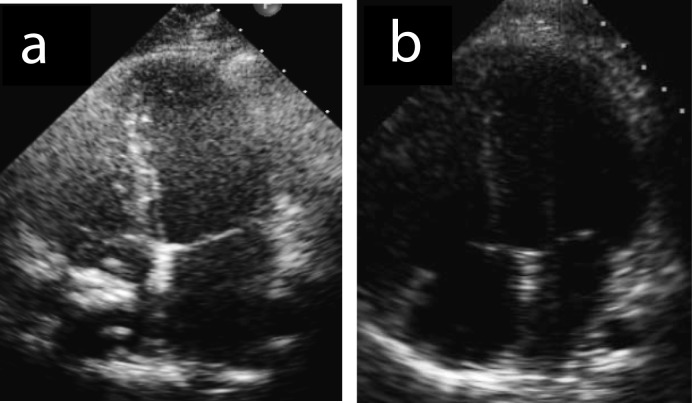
(a, b) Echocardiogramm post-surgery revealed absence of thrombus.
